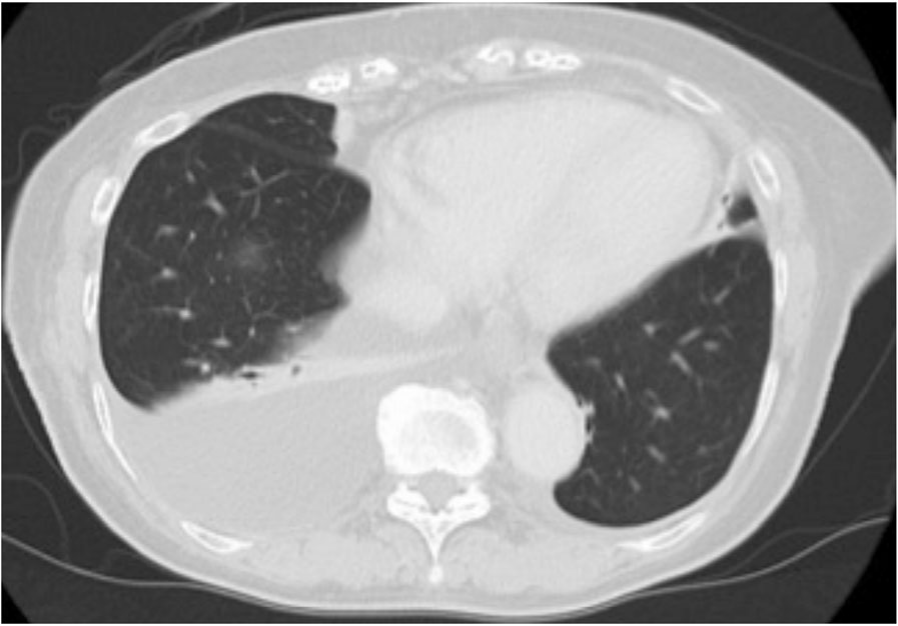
Computed tomography showing reaccumulation of pleural fluid on postoperative day 57
